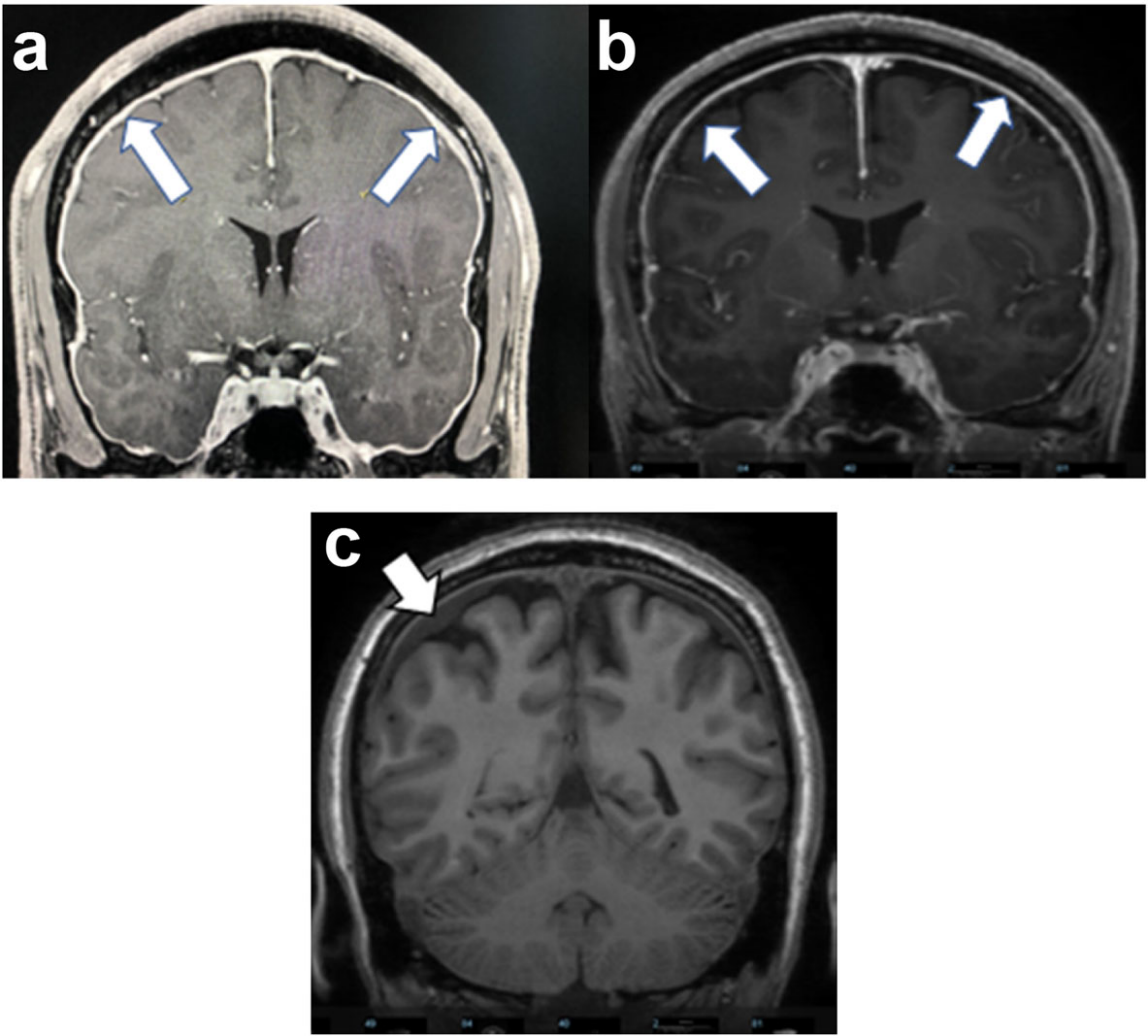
a Patient 1 b Patient 2: Magnetic Resonance Imaging with gadolinium (Coronal T1 Spoiled Recalled Gradient-Echo) demonstrated diffuse pachymeningeal enhancement (arrows), a feature of intracranial hypotension. c: Patient 2: Magnetic Resonance Imaging (Coronal T1) demonstrated subdural collections (arrow), a feature of intracranial hypotension
